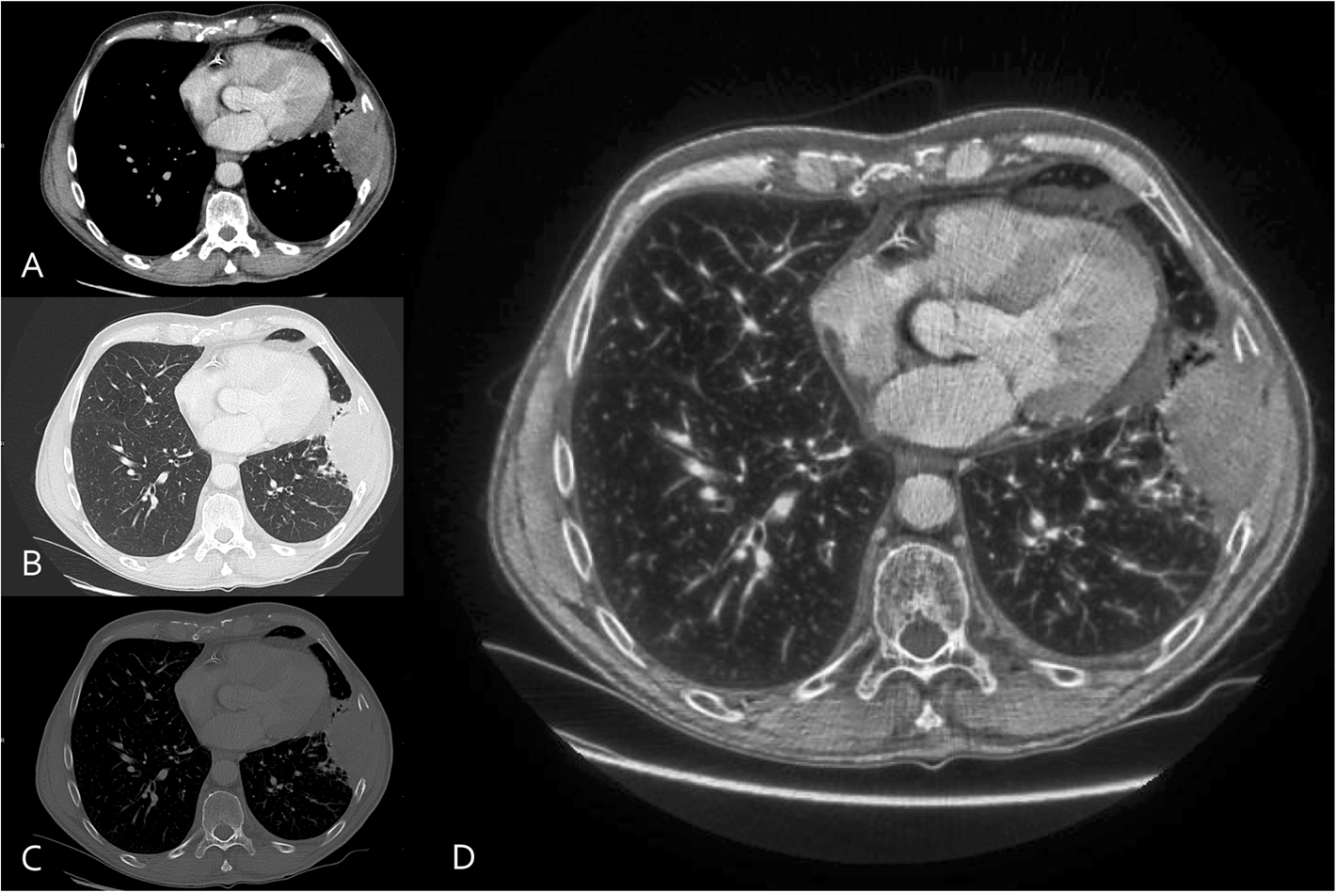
Axial contrast-enhanced CT-images (3 mm CT-reconstructions) in a 70-year-old man with stage IV lung adenocarcinoma with large mass at the left lower lobe with chest wall involvement and bone erosion. Images at the same level are shown in mediastinal (a), lung (b), and bone (c) window setting as well as a single AIO-window (d)
